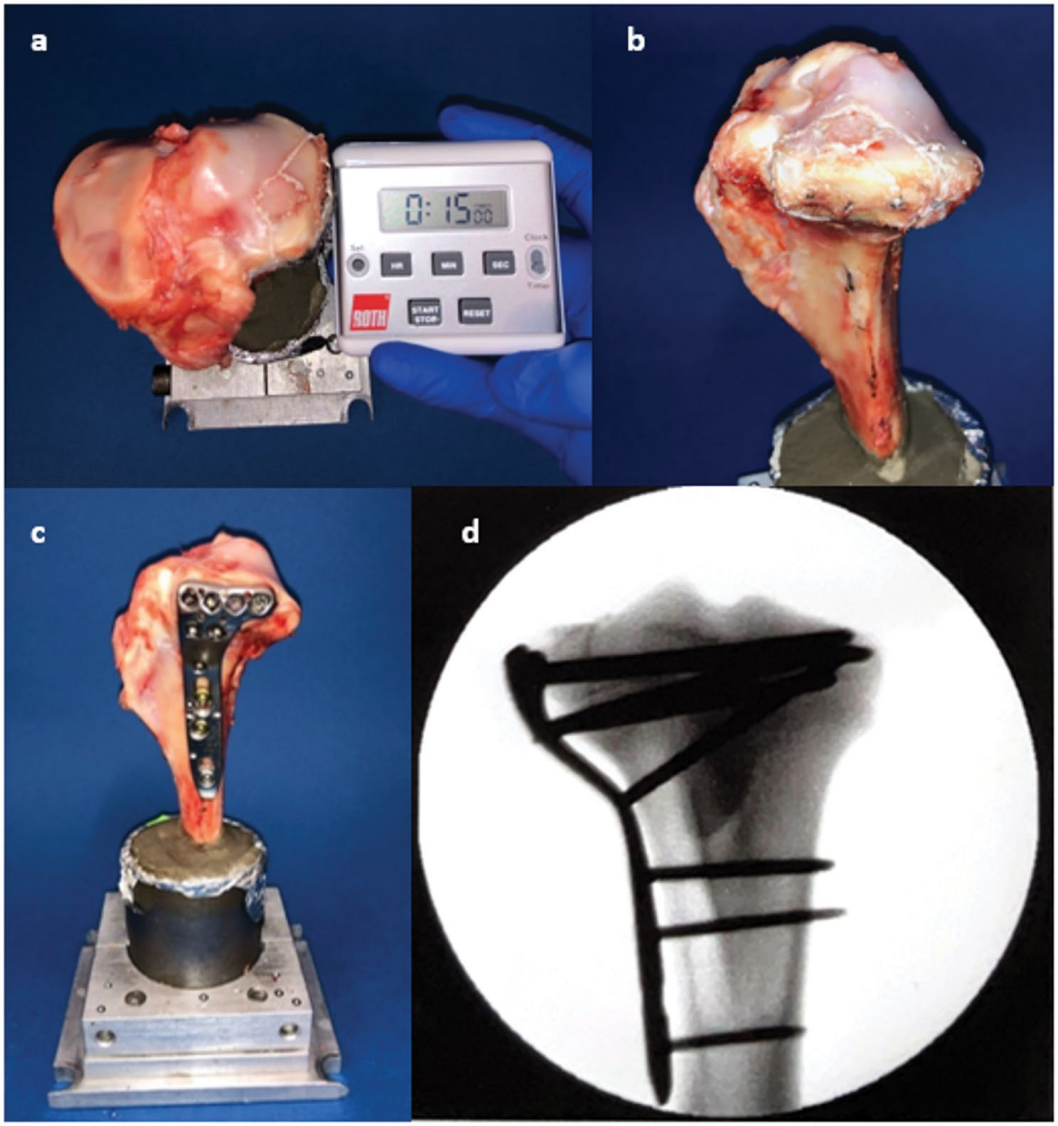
The progress of the operation technique is shown. First, the depression zone was reduced, raised to the anatomical correct level and then the bone cement with a curing phase of 15 min was applied (a + b). The fracture was then supplied with a combination of cement and plate osteosynthesis from lateral (c) and controlled radiographically (d)
